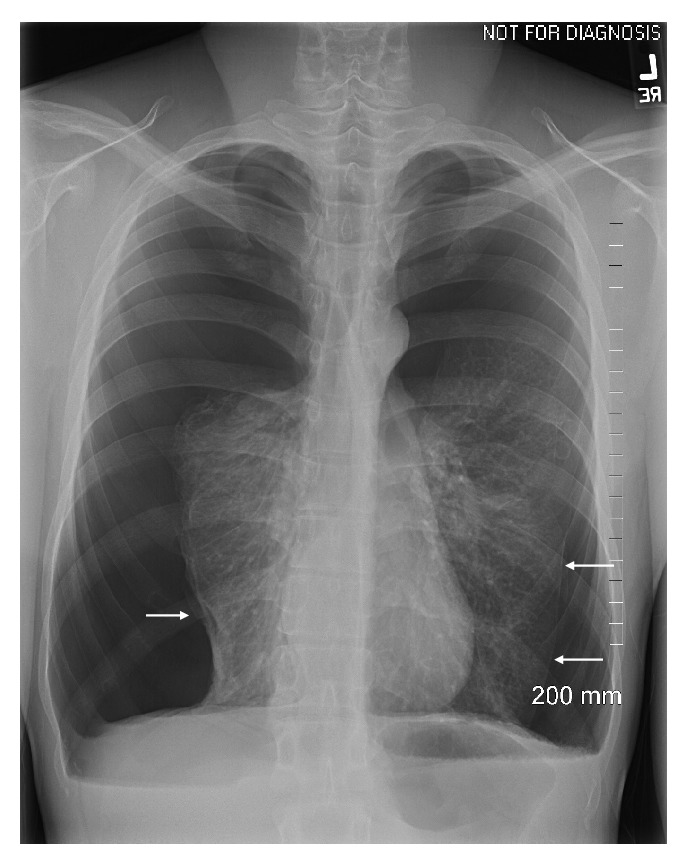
Chest radiograph with bilateral spontaneous pneumothorax at presentation. Pleural line is visible (arrow).
